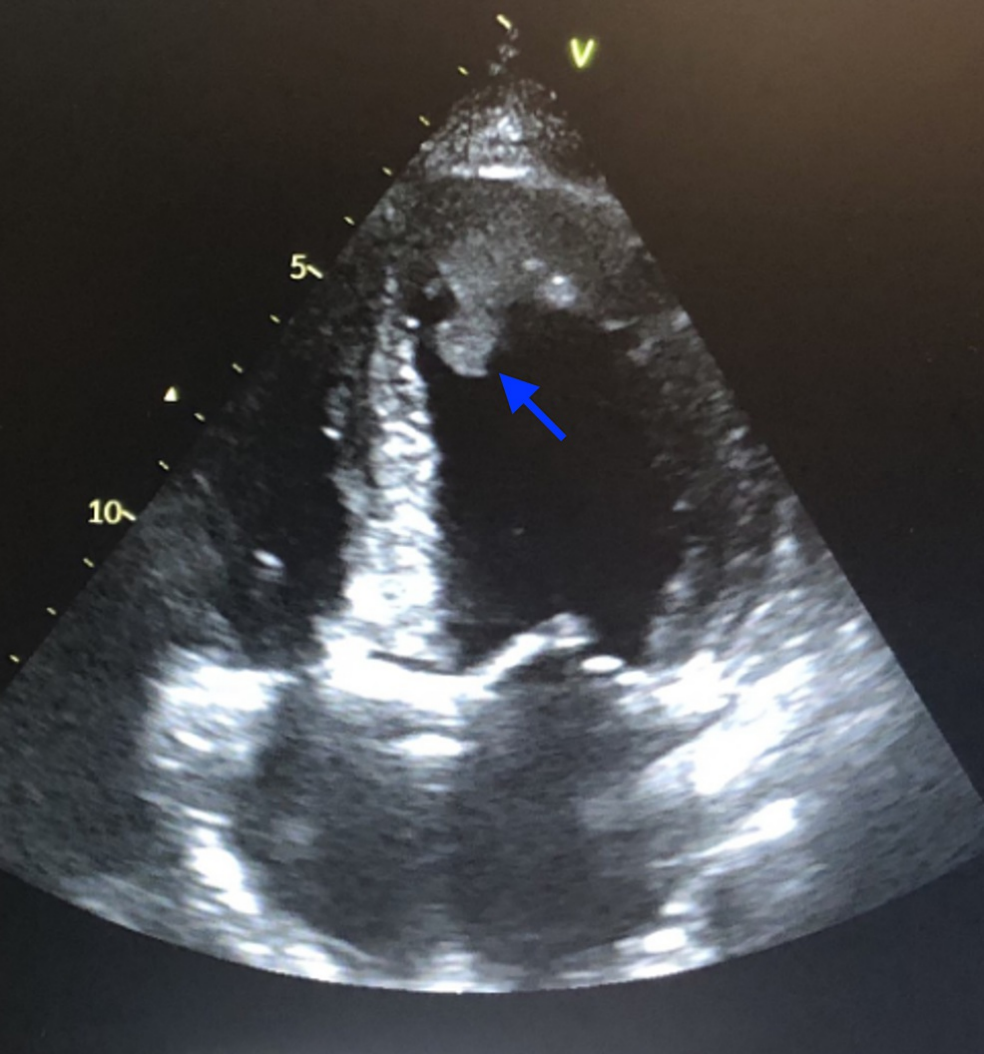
Echocardiogram showing globally enlarged ventricles with an apical thrombus in the left ventricle (blue arrowhead)
